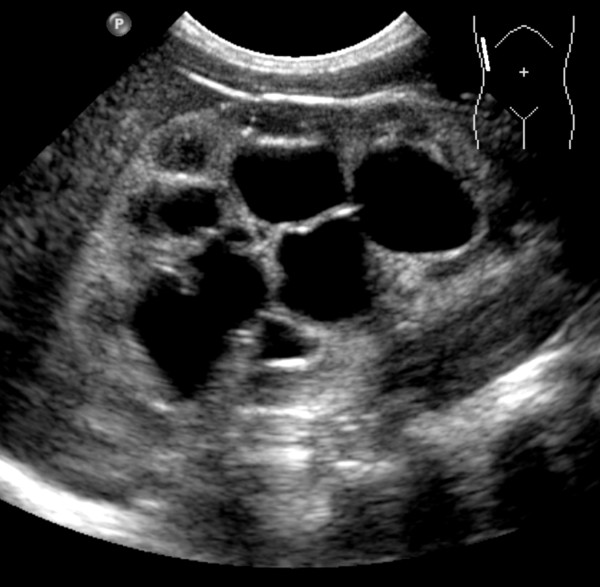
Renal ultrasound. Hyperechogenic renal parenchyma due to persistent *Candida *pyelonephritis and massive pelviectasis.
